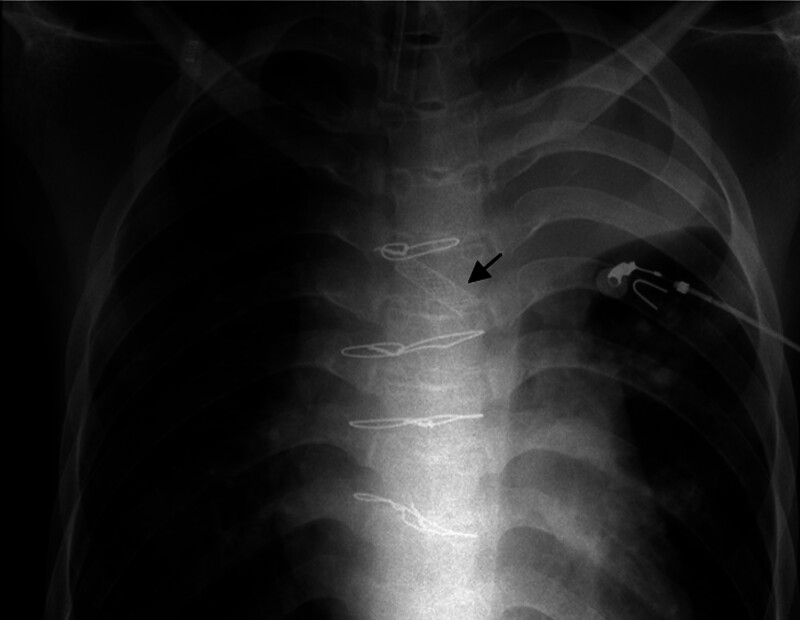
Post-stent chest X-ray showing the implanted stent (black arrow).
